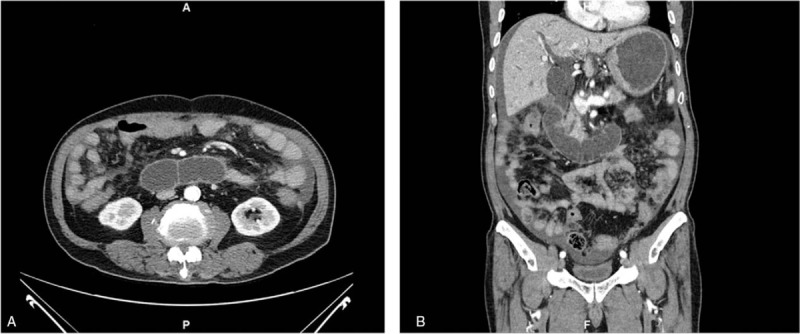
Abdominal contrast-enhanced computed tomography on admission. Images showed afferent loop obstruction (A), intrahepatic bile duct dilatation, and a large volume of ascites (B).
